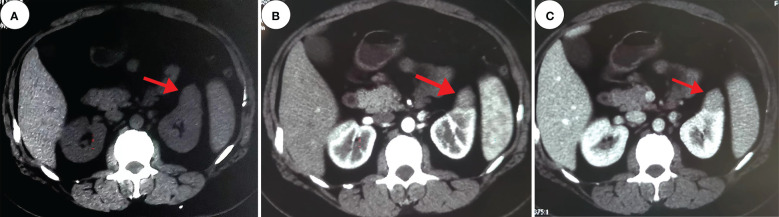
Computed tomography. These images show a 2.4 cm×2.2 cm equal-density mass in the anterior upper part of the left kidney, with poorly defined margins and a calcium density shadow in the mass [(A), red arrow]. The enhancement scan shows inhomogeneous enhancement [(B, C), red arrow].
